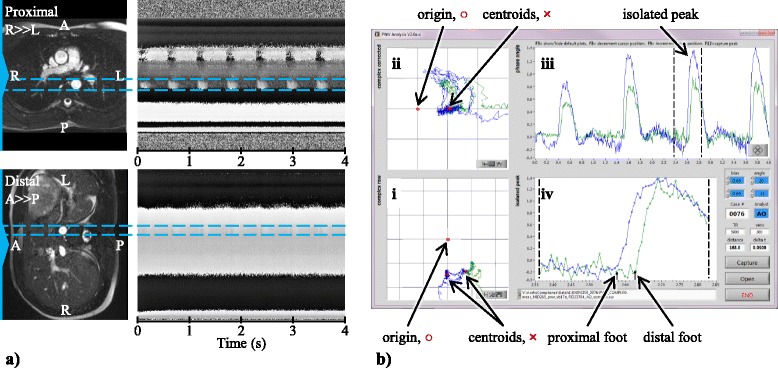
MRI PWV analysis. a Region of interest selection guided by anatomical images (*left*) and corresponding RACE phase image (*right*). b Correction for stationary tissue signal in the complex domain, with proximal (superior) slice signal in the vessel region shown in *blue* and distal (inferior) signal in *green*. b-*i* Raw data plotted in the complex plane, showing centroids marking slow flow signal. b-*ii* Complex signal relative to the reference static tissue point. b-*iii* Phase waveforms relative to the reference point. b-*iv* Estimation of foot transit time
